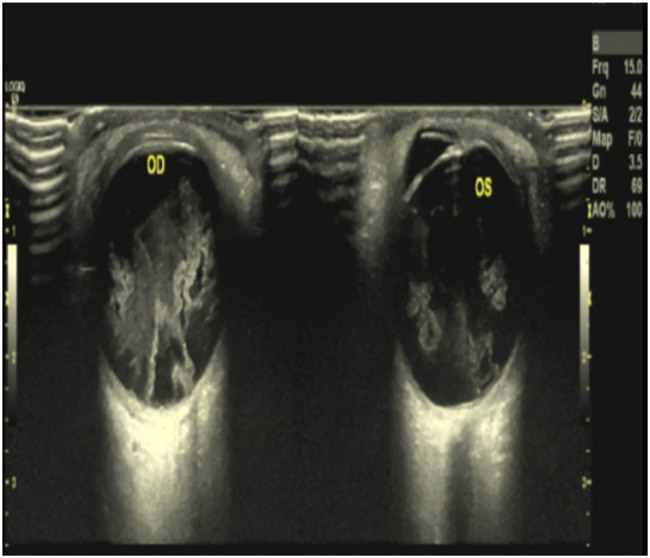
Ultrasonography of both eyes revealed vitreous opacity in both eyes.
